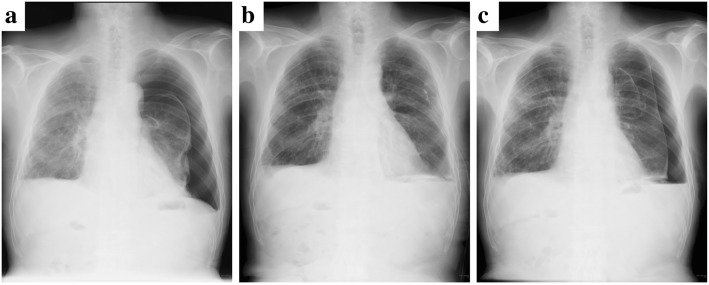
Chest radiograph showing a left pneumothorax on admission (a). The lung expansion improved after repeat pleurodesis (b). A relapsed left pneumothorax occurred on readmission (c)
